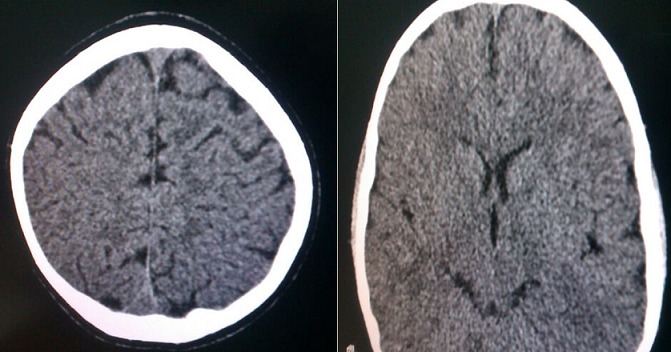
Image du CT scan montrant une résorption de l'oedème et de l'hématome
